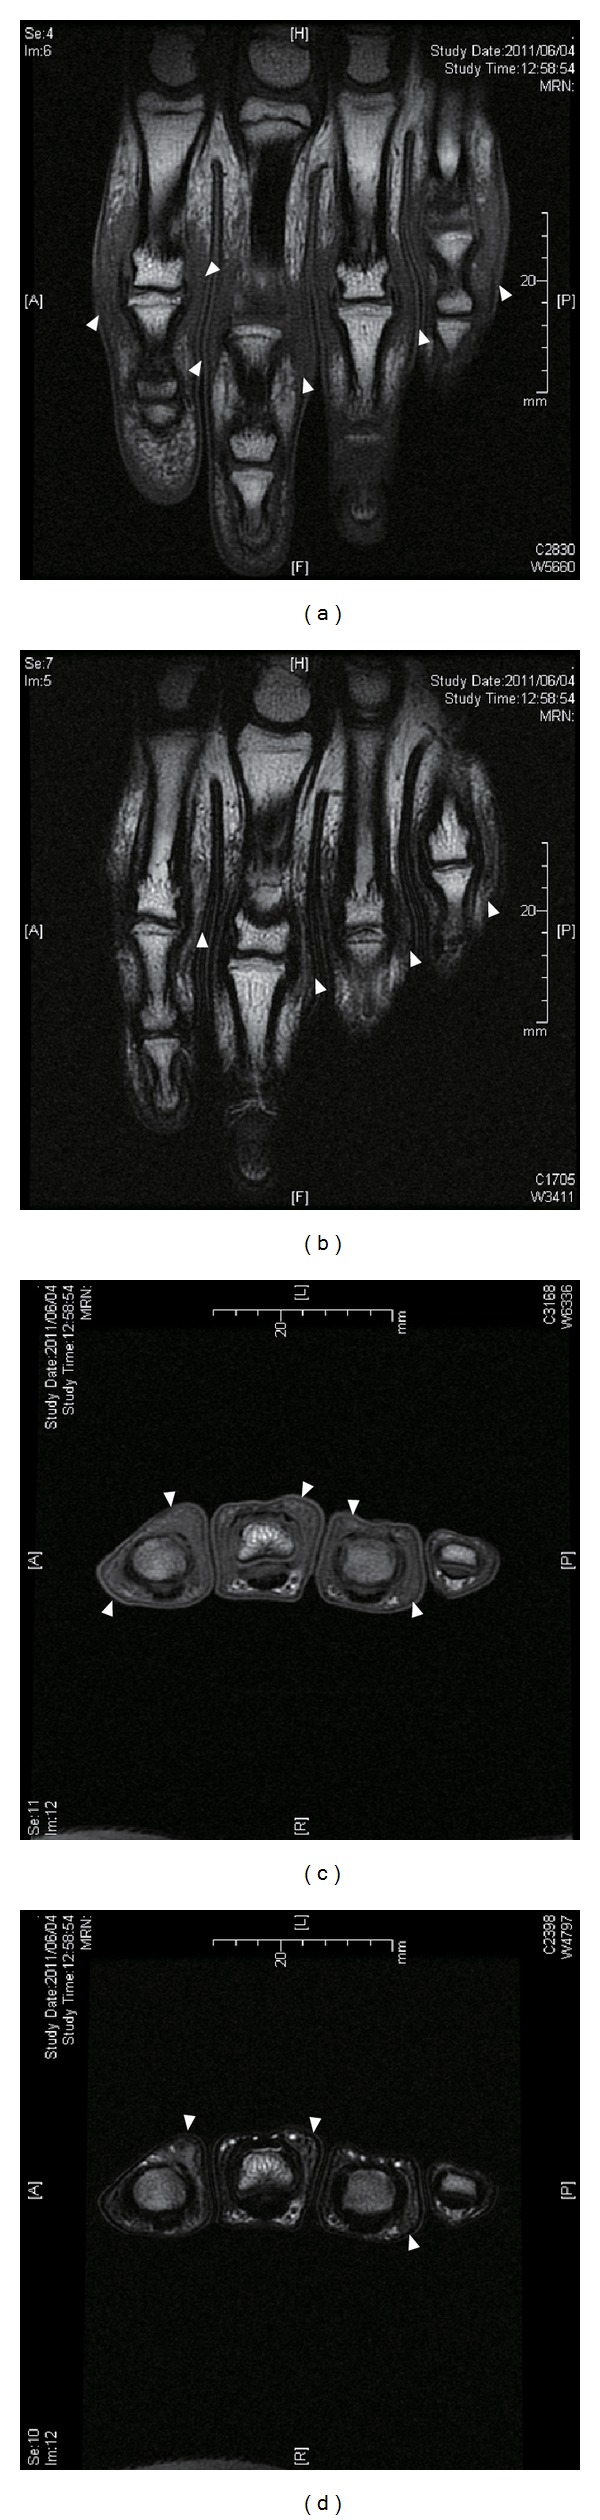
Coronal (a, b) and axial (c, d) views of magnetic resonance imaging of the left hand. Homogeneous low-intensity subcutaneous lesion around the PIP joints (arrow heads) on T1-weighted image (a, c) and T2-weighted image (b, d).
